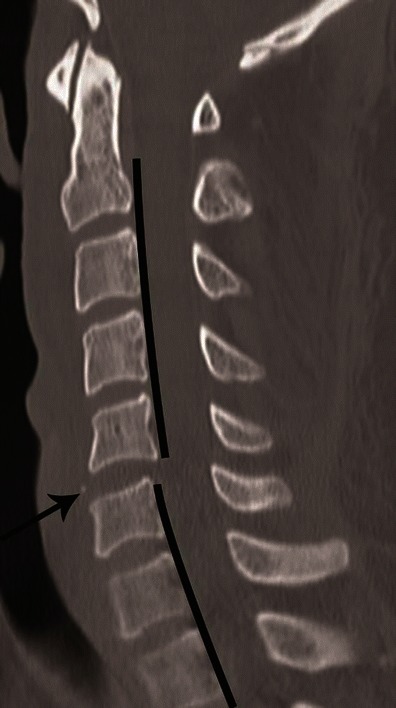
Hyperextension distraction with dislocation. Mid-sagittal CT image shows the significant prevertebral soft tissue swelling and subtle anterior widening of the C5–C6 disc space—a hallmark of hyperextension distraction injury. A small avulsion fracture (*arrow*) is noted at C5–C6. Mild retrolisthesis of C5 on C6 vertebral body suggested by disrupted posterior vertebral line (*black lines*)—suggestive of hyperextension dislocation
